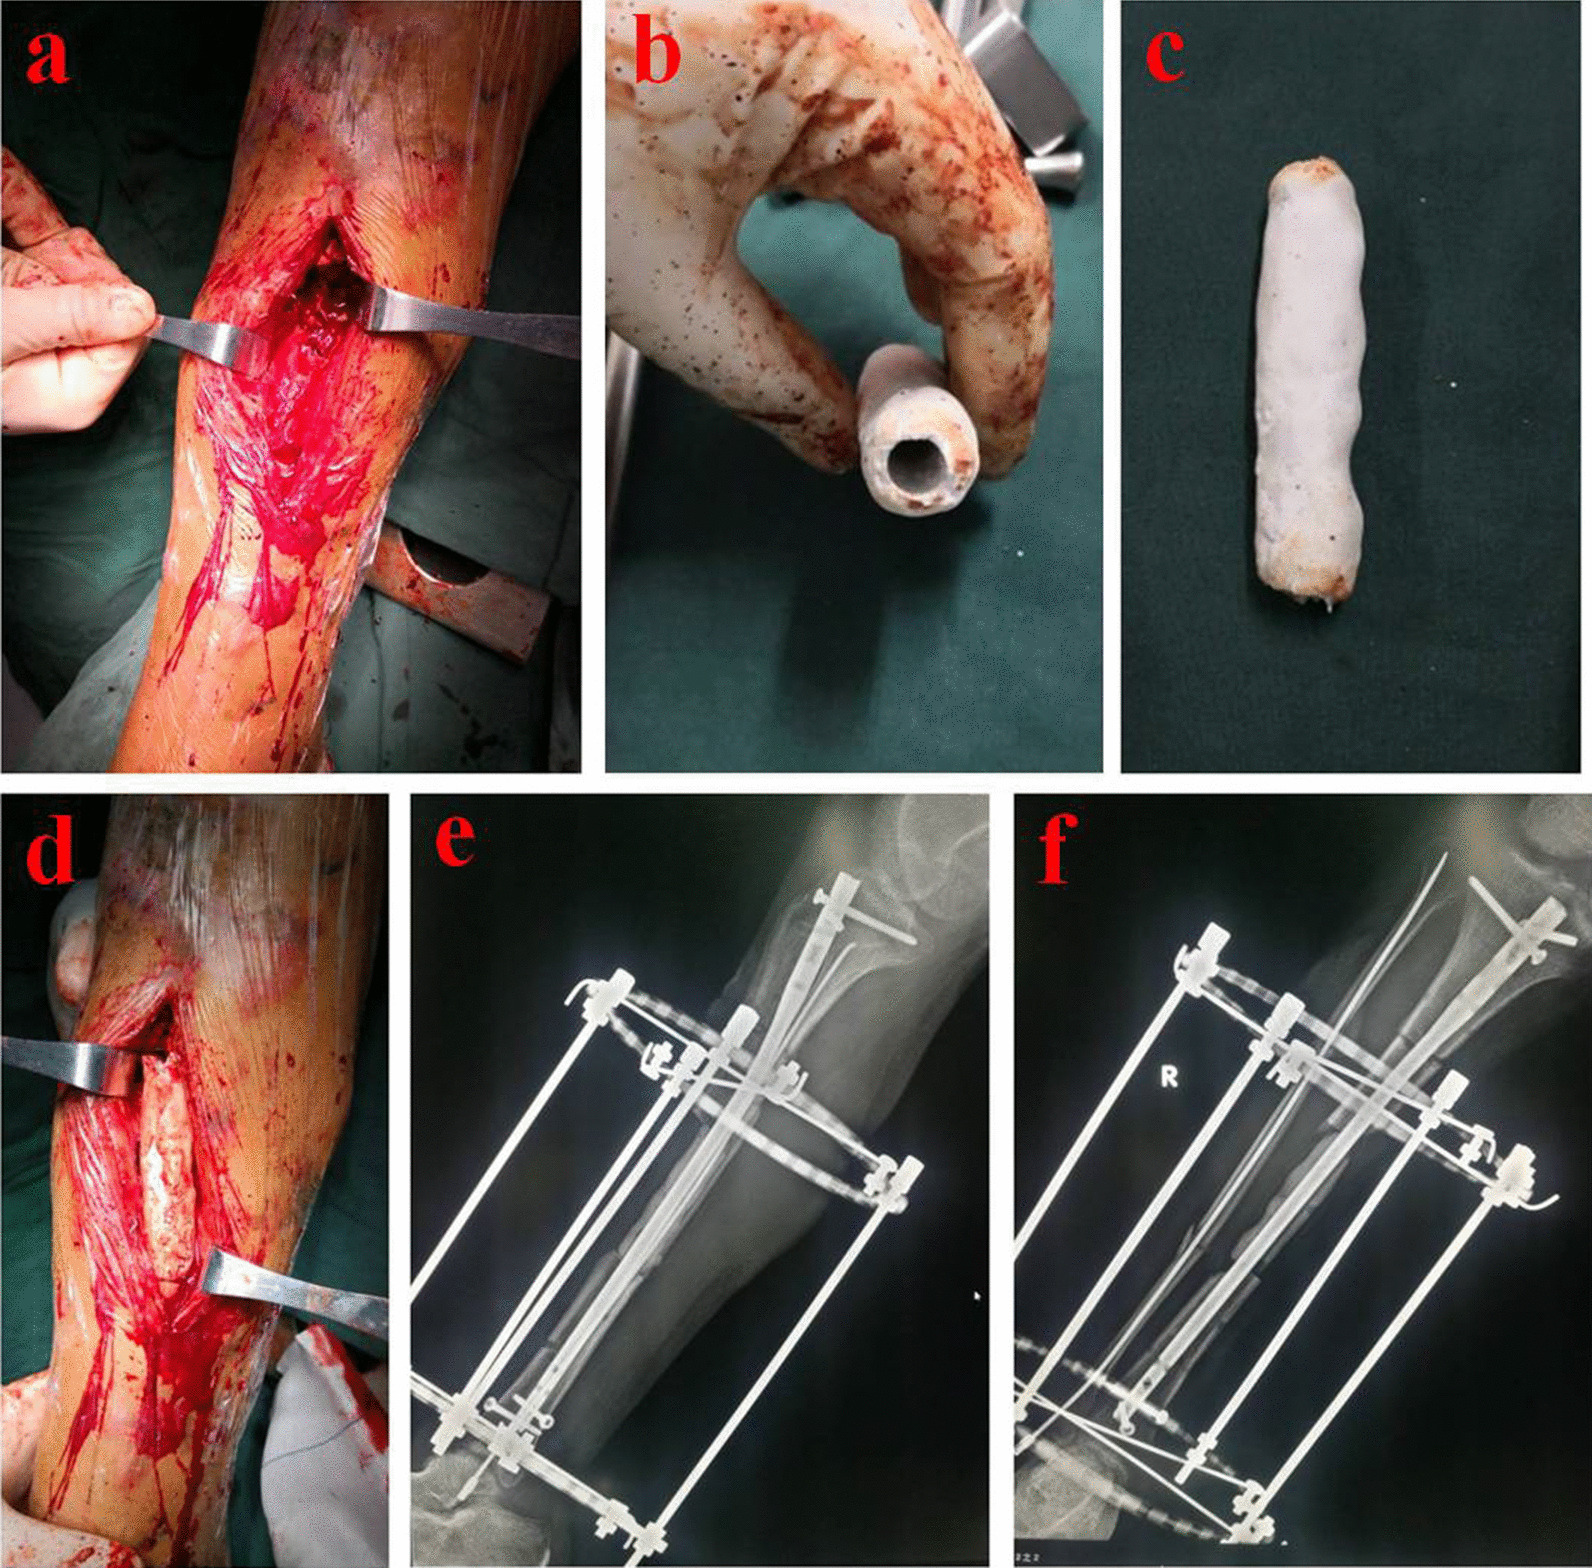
The 35-year-old patient was treated by the ACSLHT technique. a A large defects in the middle of tibia could be seen without infection, b and c an antibiotic-loaded calcium sulfate cement spacer was made during operation; d the spacer was implanted into the tibial defect area, e and f X-rays after an intramedullary nail and Ilizarov external fixator implantation. ACSLHT stands for antibiotic calcium sulfate-loaded hybrid transport
